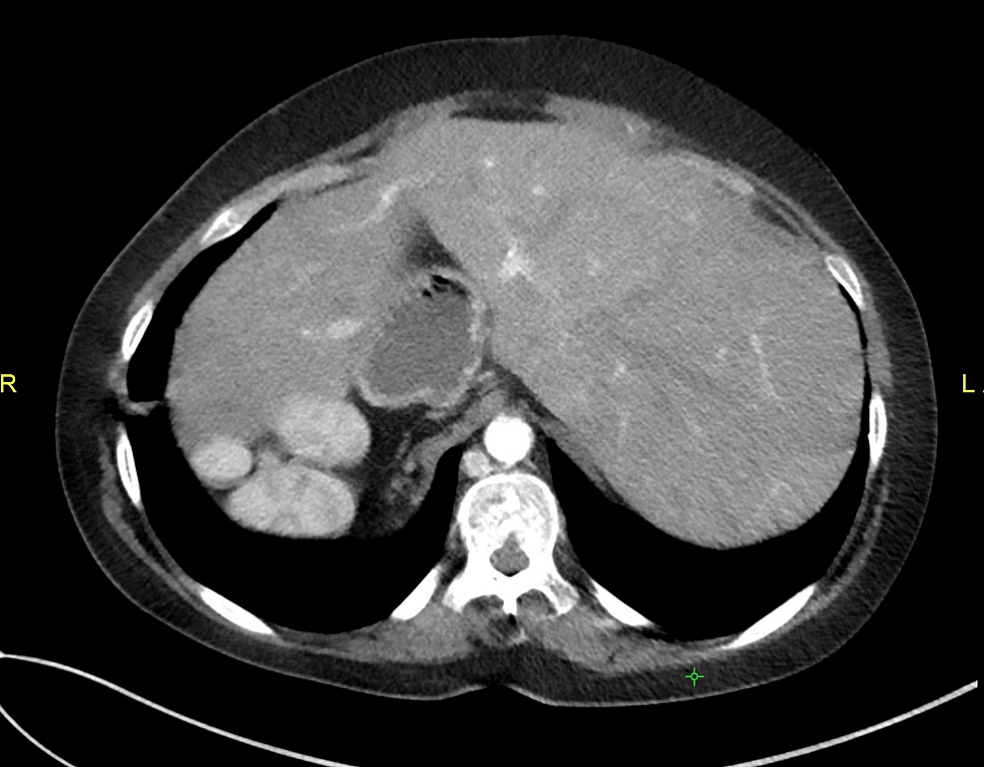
Axial abdominal CT image showing midline liver CT: computed tomography
